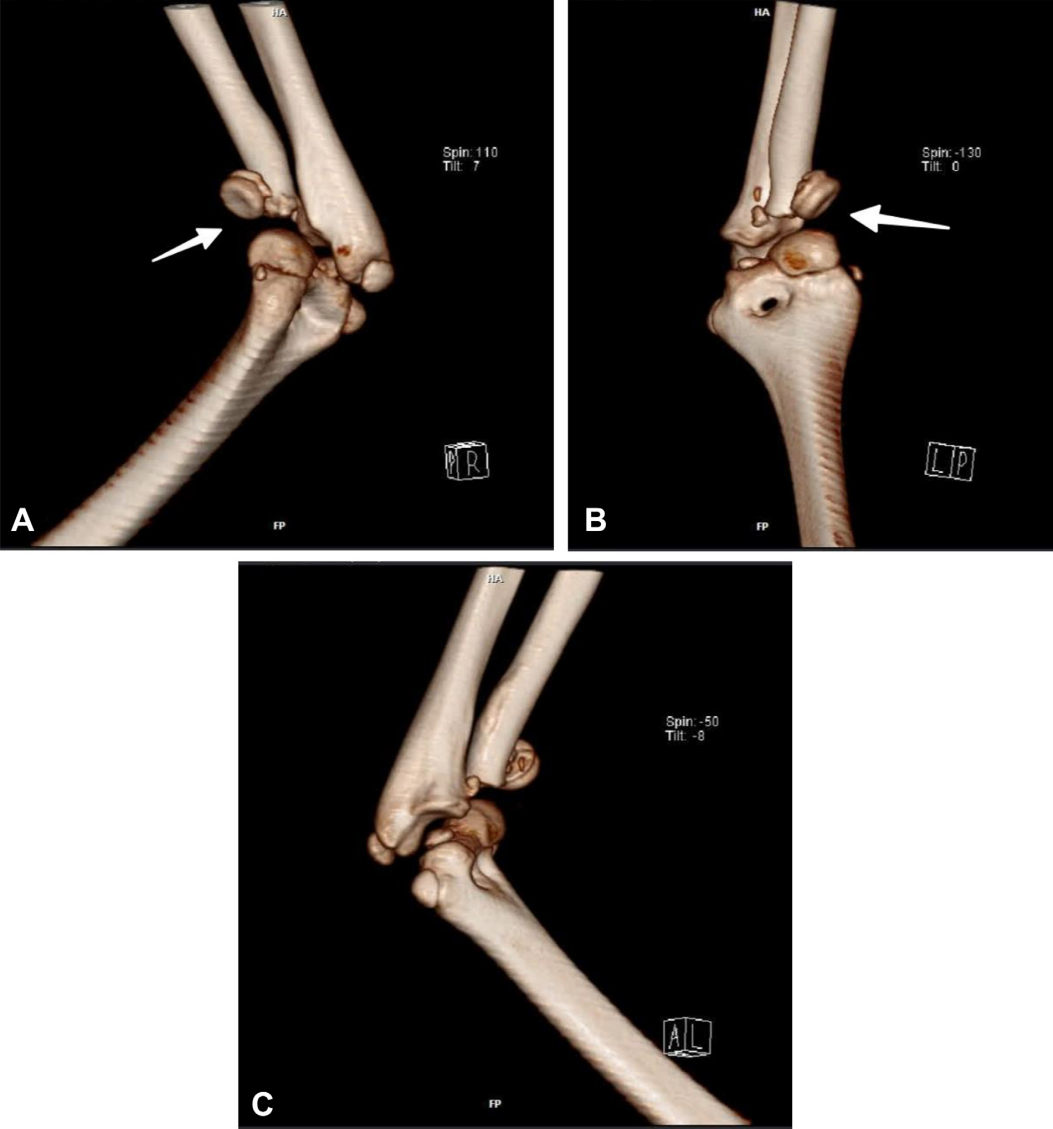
A: 3D CT reconstruction of the right elbow - lateral aspect Computed tomography with 3D volume rendering technique, lateral view, clearly demonstrating the three components of the terrible triad injury: (1) posterior dislocation of the elbow joint, (2) comminuted radial head fracture, and (3) coronoid process fracture. Note the posterior and lateral displacement of the radius and ulna relative to the distal humerus. B: 3D CT reconstruction of the right elbow - anterior aspect Computed tomography with 3D volume rendering technique, anterior view, providing enhanced visualization of the radial head comminution and coronoid process fracture (arrow). This perspective clearly demonstrates the anterior structural damage associated with the posterior dislocation mechanism. C: 3D CT reconstruction of the right elbow - medial aspect Computed tomography with 3D volume rendering technique, medial view, showing the relationship between the coronoid process fracture and the overall joint displacement. Note the integrity of the medial epicondyle despite the significant forces involved in this injury pattern.
